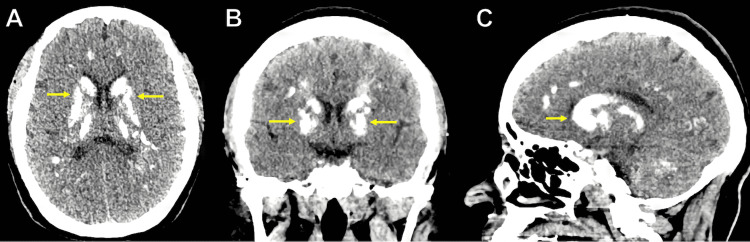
Unenhanced Brain CT scan (A) Axial, (B) coronal, and (C) sagittal reconstructions showing calcified basal ganglia (yellow arrows).
